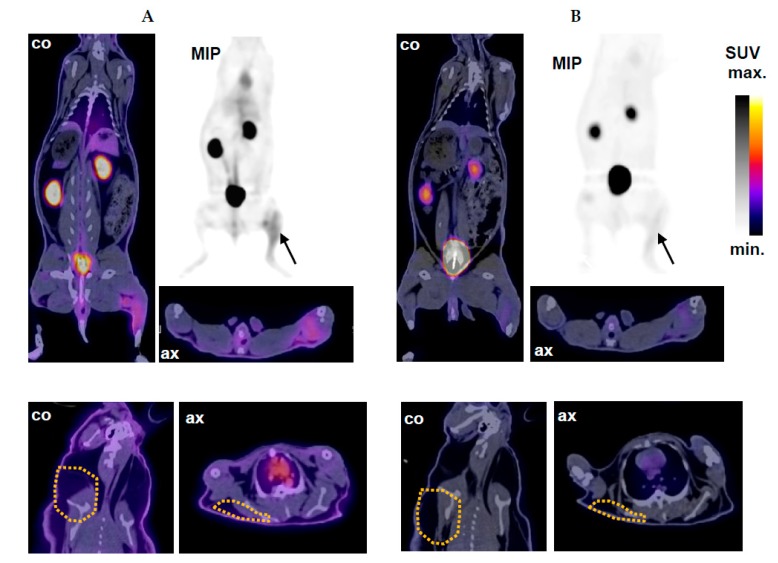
PET/CT imaging of NZR3 at (A) 5 min and (B) 60 min after injection of ^68^Ga-DOTA-TBIA101. Top panel: the MTB infection was successfully delineated by the tracer. Arrows highlight the infection site in a representative midplane-coronal (co) and axial (ax) PET/CT slice and in a maximum intensity projection (MIP) PET image. Bottom panel: A viable staphylococcal infection site in the scruff area (yellow-dotted lines) was not localized by PET/CT imaging as presented in coronal (co) and axial (ax) PET/CT slices.
